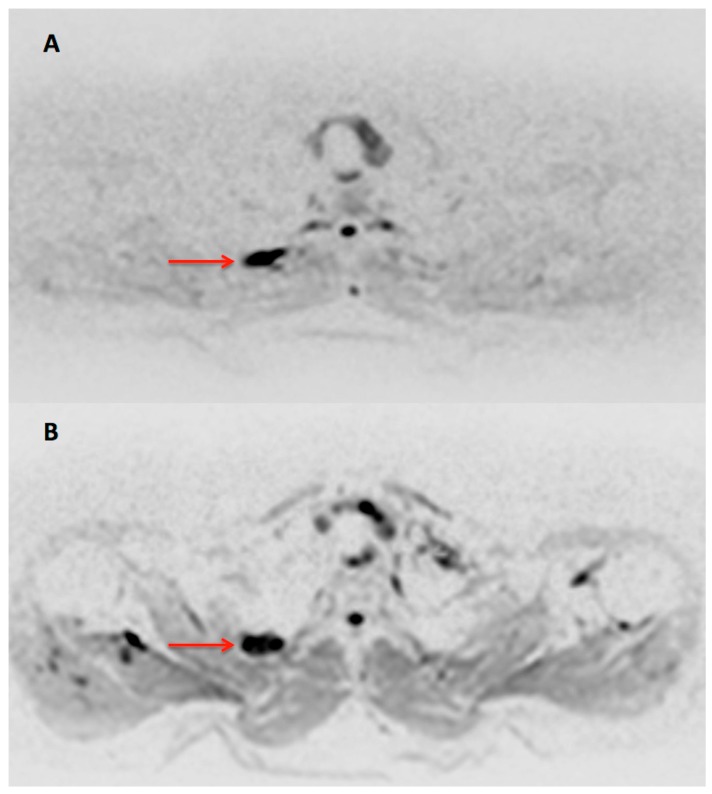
Transaxial DWI b800 (black/white inverted) images before (A) and after (B) three cycles of the combination treatment. DWI demonstrated a persistent signal elevation in the osteolytic lesion of the second rib (arrow) after the administration of treatment, despite the patient’s serological response. Although existing data are rather limited, DWI is considered a repeatable, quantifiable magnetic resonance imaging-technique that can potentially help differentiate responders from nonresponders in myeloma bone disease [9,10,11]. In an study involving a mixed population of primary and pre-treated MM patients DWI was found to be more sensitive than ^18^F-FDG PET in detecting myeloma lesions, when using the well-established imaging standard of MRI and/or CT as reference. However, this mismatch between PET and DWI findings was mainly seen in the already treated patients, who had reached at least near complete response, raising, thus, the suspicion of false-positive findings on DWI [12]. A possible explanation is the fact that diffusion imaging is based on T2-weighted sequences, and diffusion weighting is introduced using de- and rephrasing preparation gradients. As a result, strongly T2-hyperintense tissue will appear hyperintense on plain DWI-images (T2-shinethrough), as it was in the presented case. After successful treatment, solid myeloma foci often develop high signal intensity on T2-weighted images, which explains why DWI may be false positive in pre-treated myeloma. The problem can theoretically be overcome by calculating the apparent diffusion coefficient (ADC) from at least two DWI sequences using different preparation gradients. ADC maps, however, are often degraded by artefacts in areas with magnetic field inhomogeneities and respiratory motion. This was the case here, where the lesion was located in the upper thoracic aperture. On the other hand, in that study the two functional modalities (PET, DWI) were equally sensitive in the sub-population of primary, untreated MM patients [12]. This is the first case demonstrating the potentially superior performance of ^18^F-FDG PET/CT over DWI in early response evaluation of combined treatment of a monoclonal antibody, an immunomodulatory drug and dexamethasone in MM. Further prospective studies focusing on the direct comparison between PET and DWI in MM treatment response evaluation are required to elucidate this topic.
